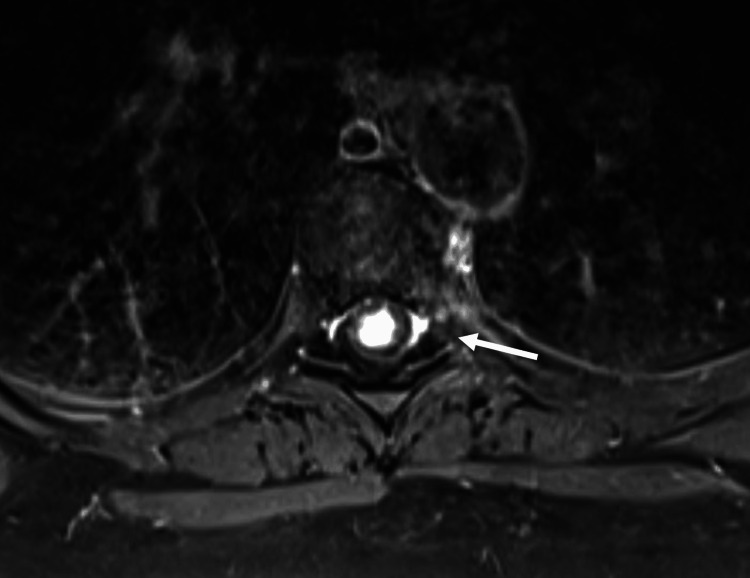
Axial MRI T1 sequence with gadolinium contrast demonstrating the same T6-7 lesion.
